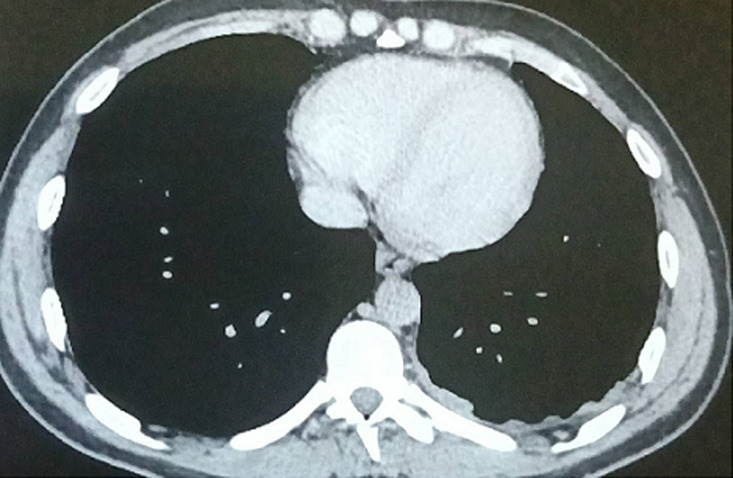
thorax computed tomography showing left-sided pleural thickening
